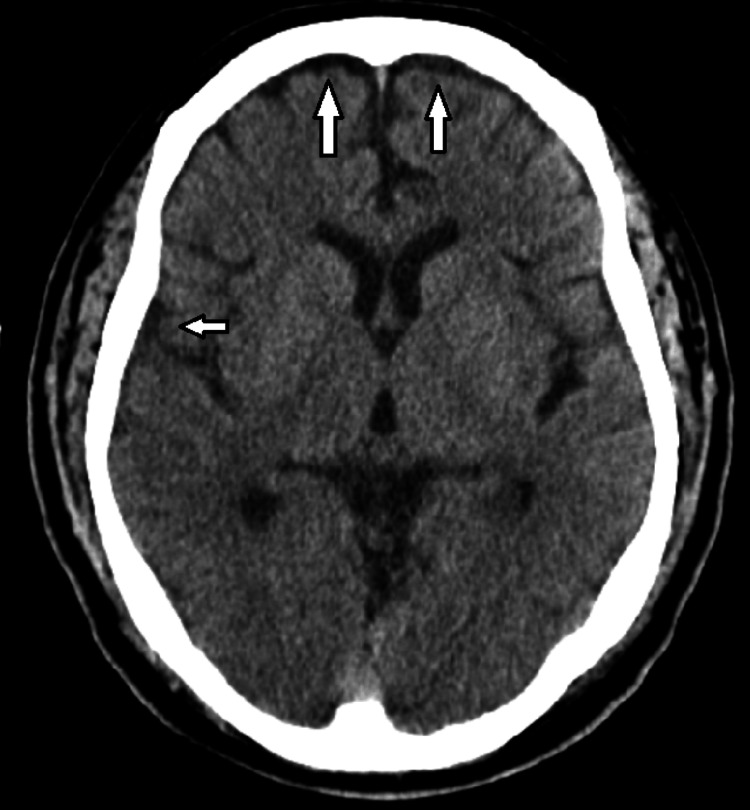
Computed tomography of the head showed mild diffuse chronic early senescent changes (white arrows).
